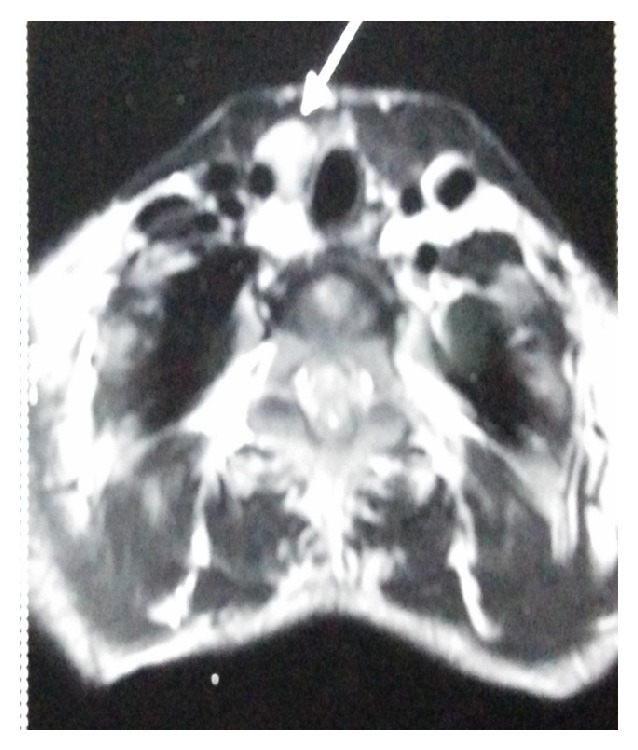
MRI showing lobulated lesion at the inferolateral aspect of right lobe of thyroid gland.
